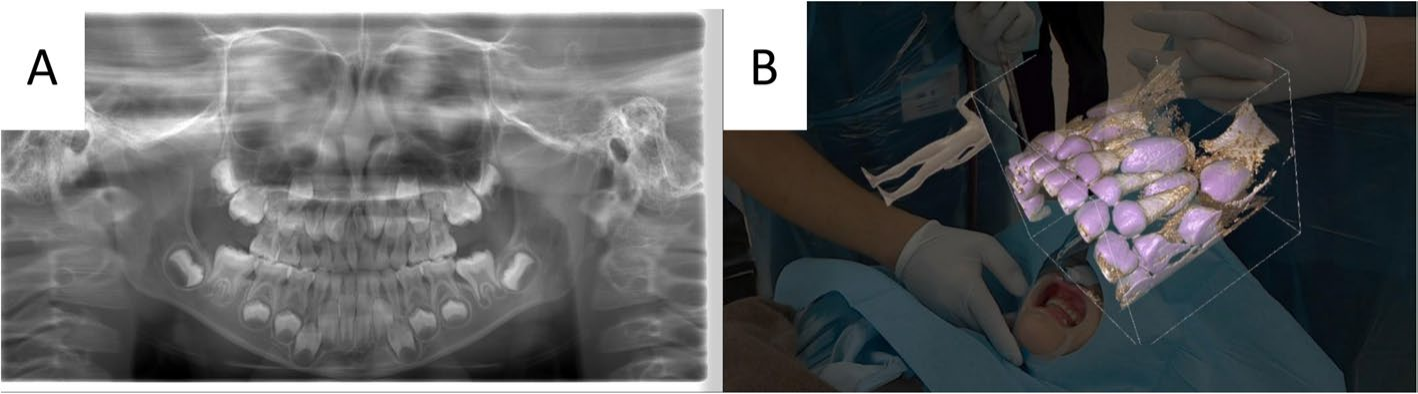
Case 3. A Panoramic radiograph. B Intraoperative view using MR technology
